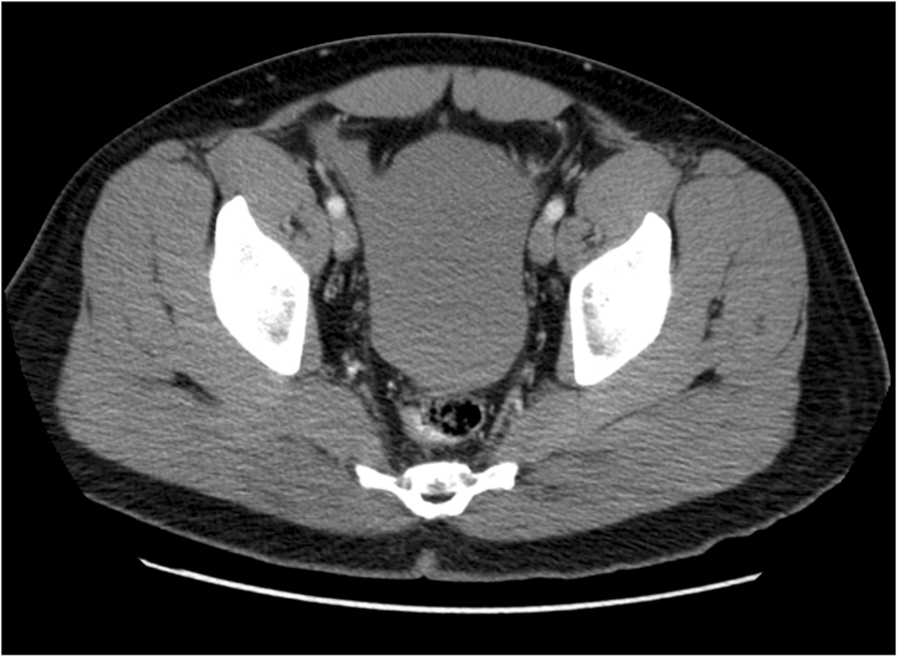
Axial, contrast-enhanced CT image of the pelvis demonstrates large hemoperitoneum.
